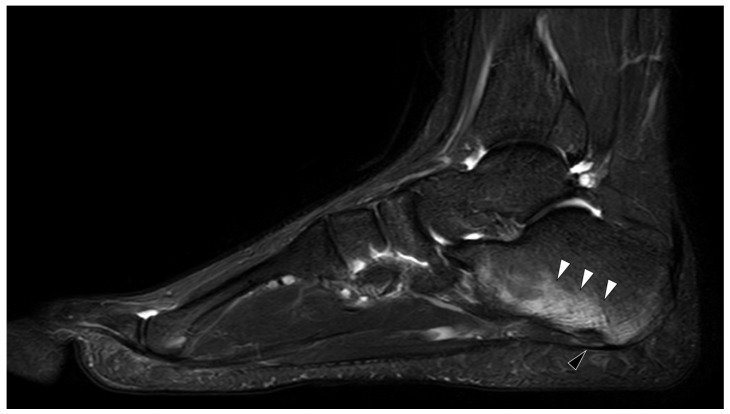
MRI in Familial Mediterranean fever (FMF). Sagittal T2 weighted with fat saturation image of an ankle of an 18 years old male with known FMF and exertional leg pain. There is characteristic enthesitis (black arrowhead) with extensive calcaneal bone marrow edema (white arrow heads) at the insertion of the long plantar tendon.
